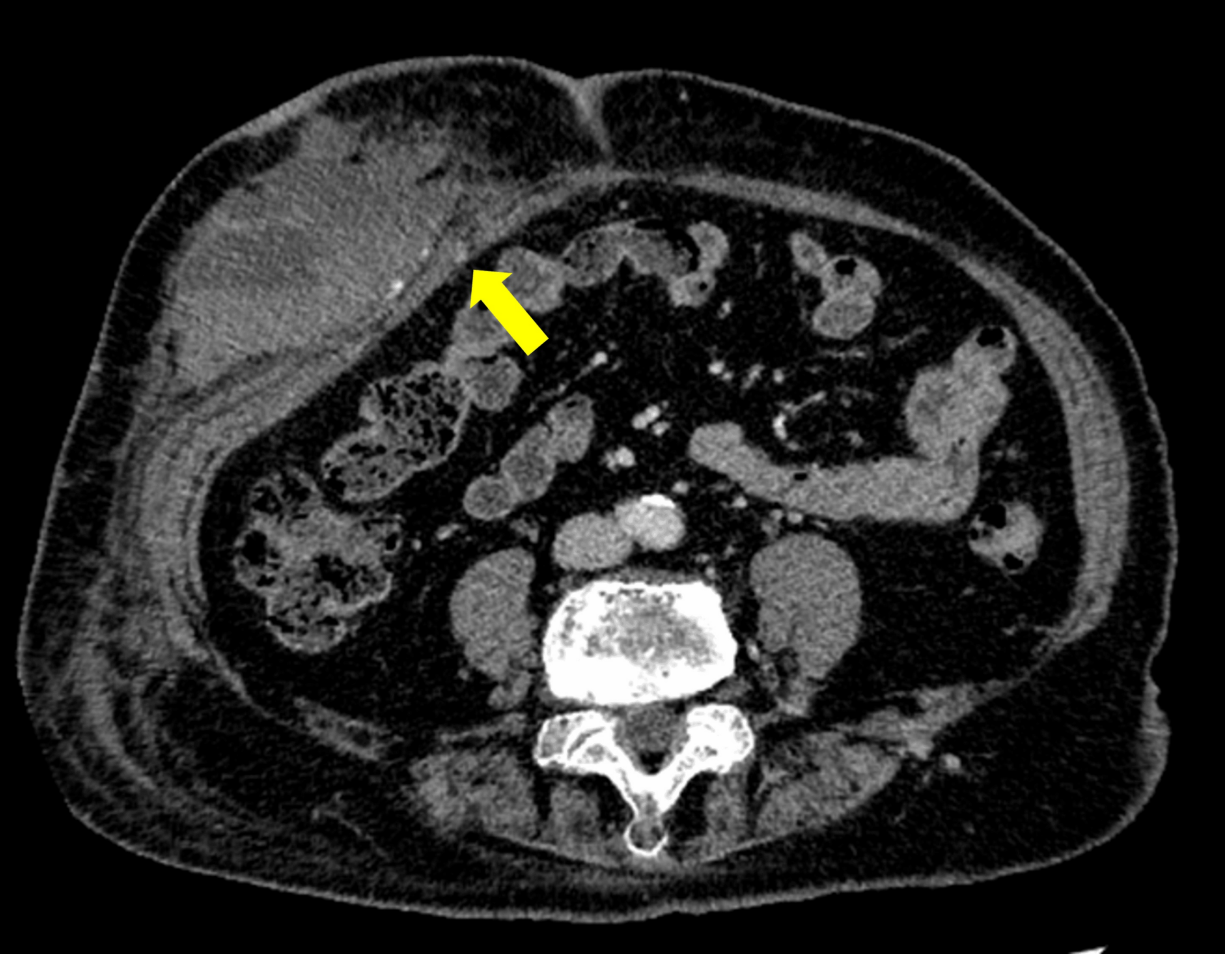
Contrast-enhanced CT showing bleeding from a perforating branch of the inferior epigastric artery
